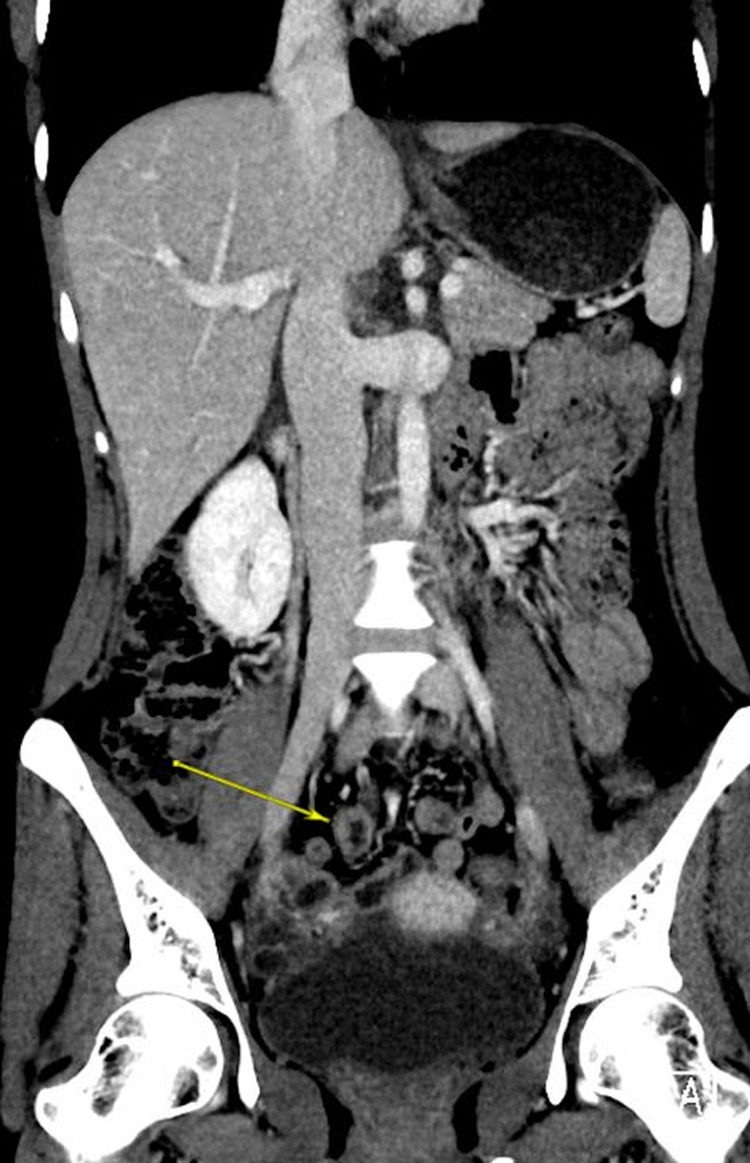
Initial abdominal CT showing multiple segmental thickening of the small bowel wall (yellow arrow)
